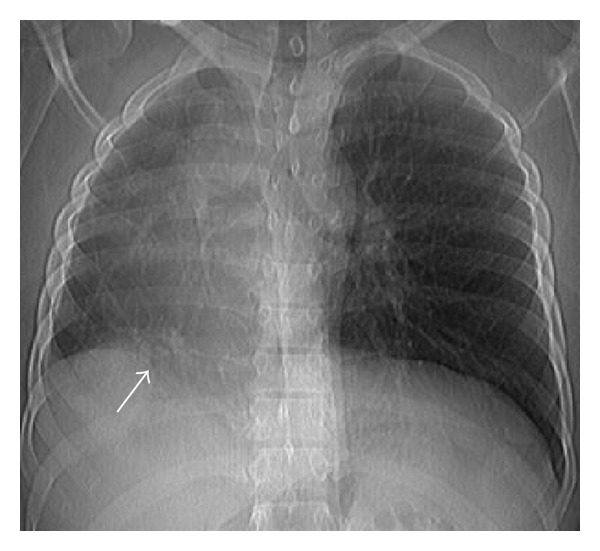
Chest radiograph depicts consolidation at right lower zone (white arrow) in addition to reduced right lung volume and ipsilateral shifting of cardiac shadow.
